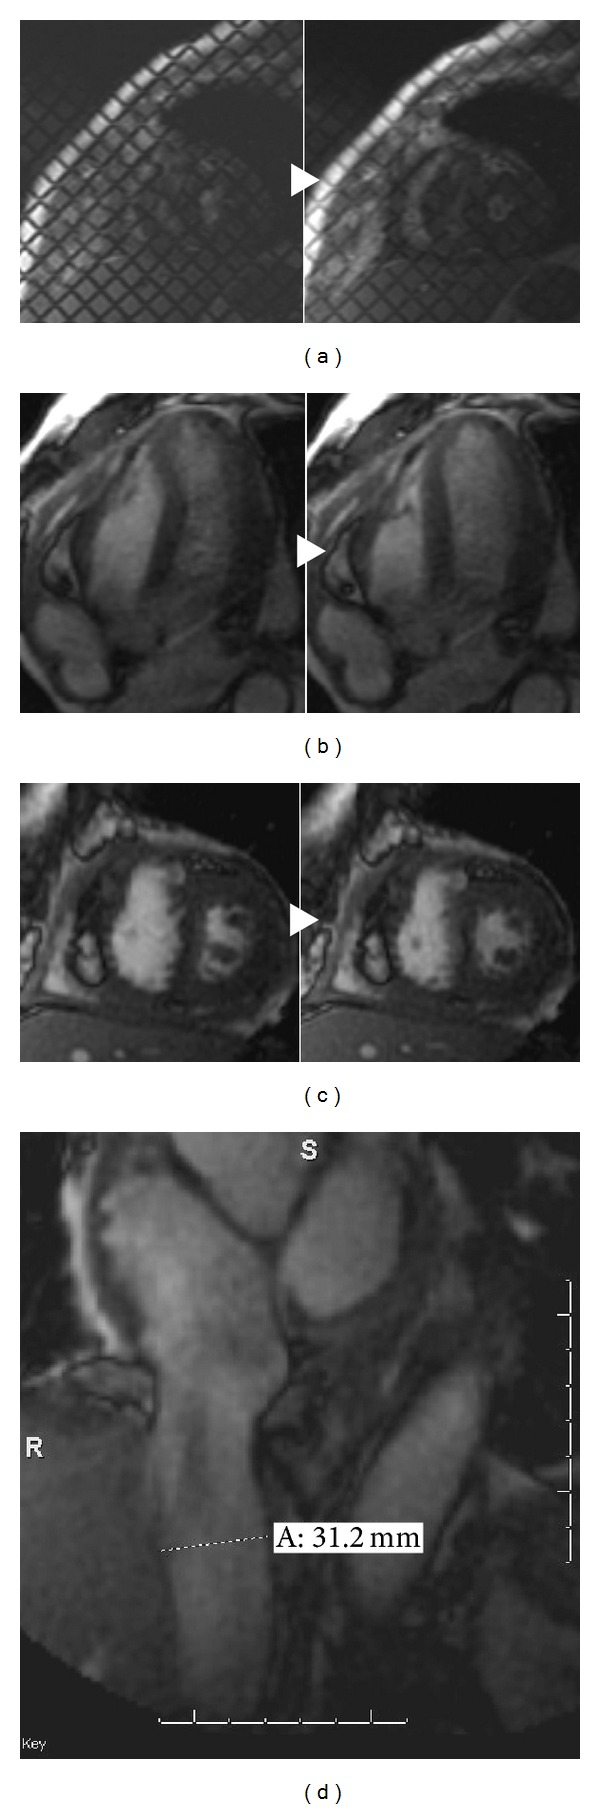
Constrictive pericarditis. A 67-year-old male with seropositive rheumatoid arthritis. An echocardiogram (images not available) was performed showing pericardial thickening and a small effusion. Paired images a, b, and c represent static images from dynamic MR imaging sequences. Cine tagged MR imaging: Transient fiducial grid-patterned image markers on sagittal images (a) demonstrates failure to dephase after several seconds, indicating nonslippage. Had the pericardium moved with respect to myocardium, the tag lines would have been broken. Instead they deformed only slightly, indicative of pericardial adhesion and providing evidence for constriction. (b, c) Breath-held SSFP long and short axis images demonstrate “septal bounce.” (d) The inferior vena cava is distended at 3.1 cm, providing corroborating evidence for elevated right heart pressures [30].
